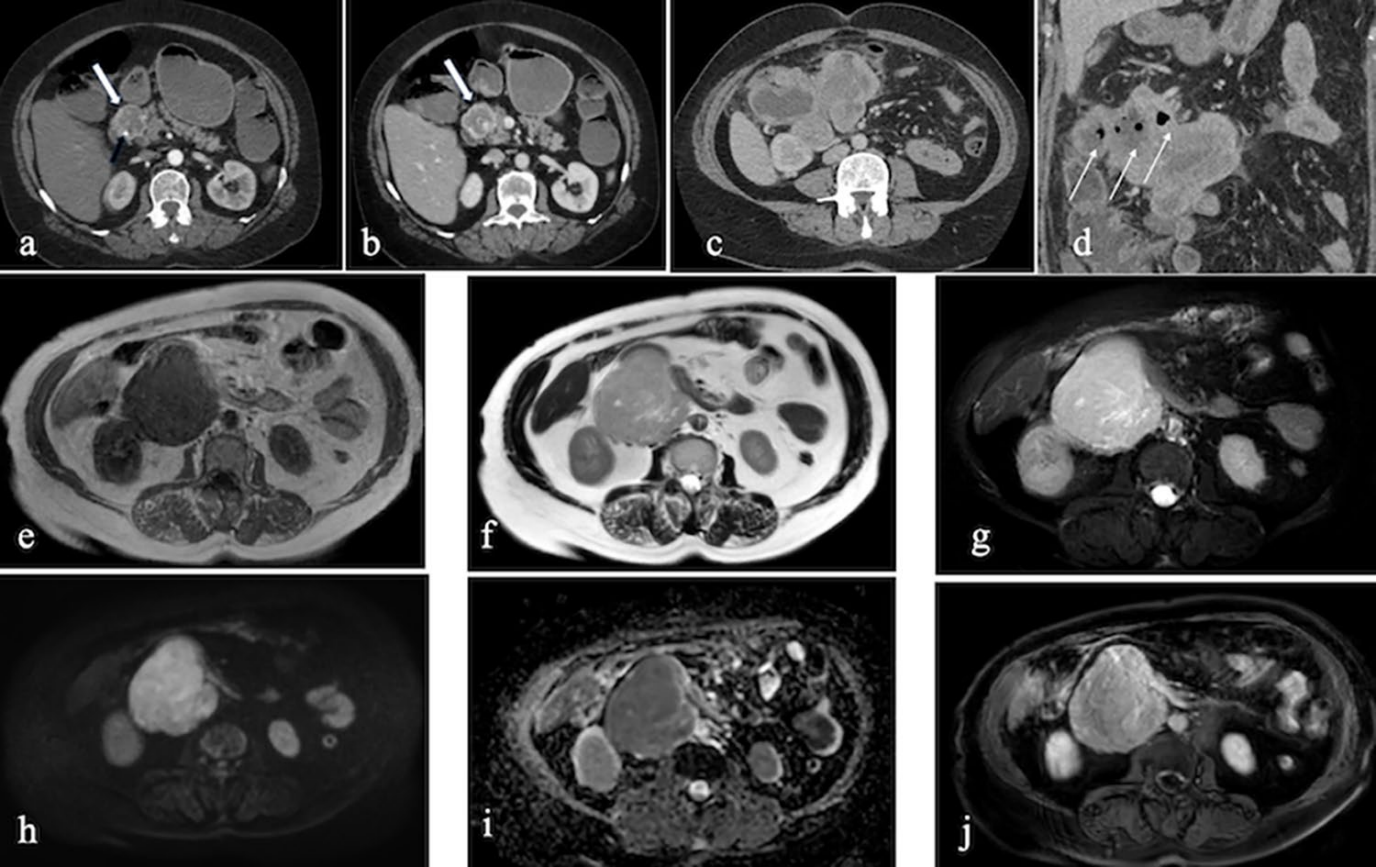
Axial IV contrast-enhanced CT images of two patients with duodenal GISTs: a, b A 50-year-old male patient with a well-demarcated hypervascular lesion containing focal calcifications (thick arrow). IV contrast-enhanced c. Axial, d. Coronal CT images of a 67-year-old male patient with a larger lesion containing air densities, indicative of necrosis (thin arrow). e. Axial T1-weighted, b. Axial T2-weighted, c. Axial fat saturated T2-weighted, d. Diffusion weighted, e. ADC maps and f. Contrast enhanced fat saturated T1-weighted MRI images of a 58-year-old female patient diagnosed with GIST, demonstrating hypervascularity, diffusion restriction, and heterogeneous enhancement
